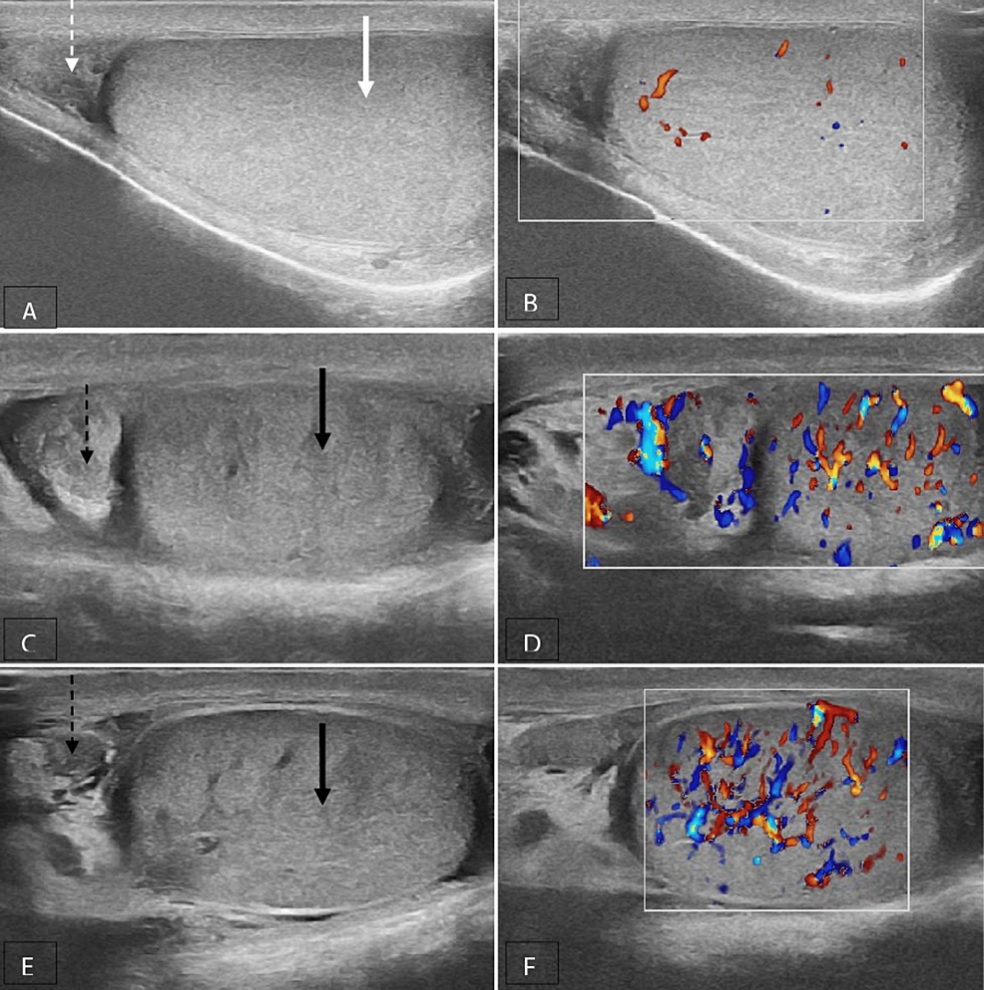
Acute epididymo-orchitis A, B Grey-scale and colour Doppler images of a normal right testis (solid white arrow) and epididymis (dotted white arrow); C, D, E, F Grey-scale and color Doppler images of the left testis (solid black arrow) and epididymis (dotted black arrow), which are enlarged in size and show heterogeneous echotexture with increased vascularity.
